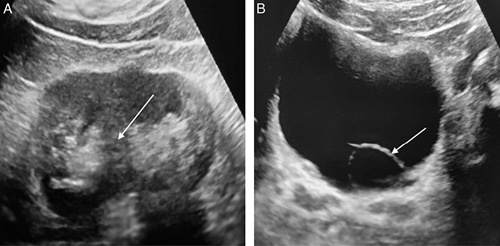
(A) Gray scale ultrasound image showing echogenic renal pelvis separated by the renal cortex in between (white arrow). No dilataion of renal pelvis seen. (B) Gray scale ultrasound image showing the cystic dilatation of intravesical part of left distal ureter (white arrow).
